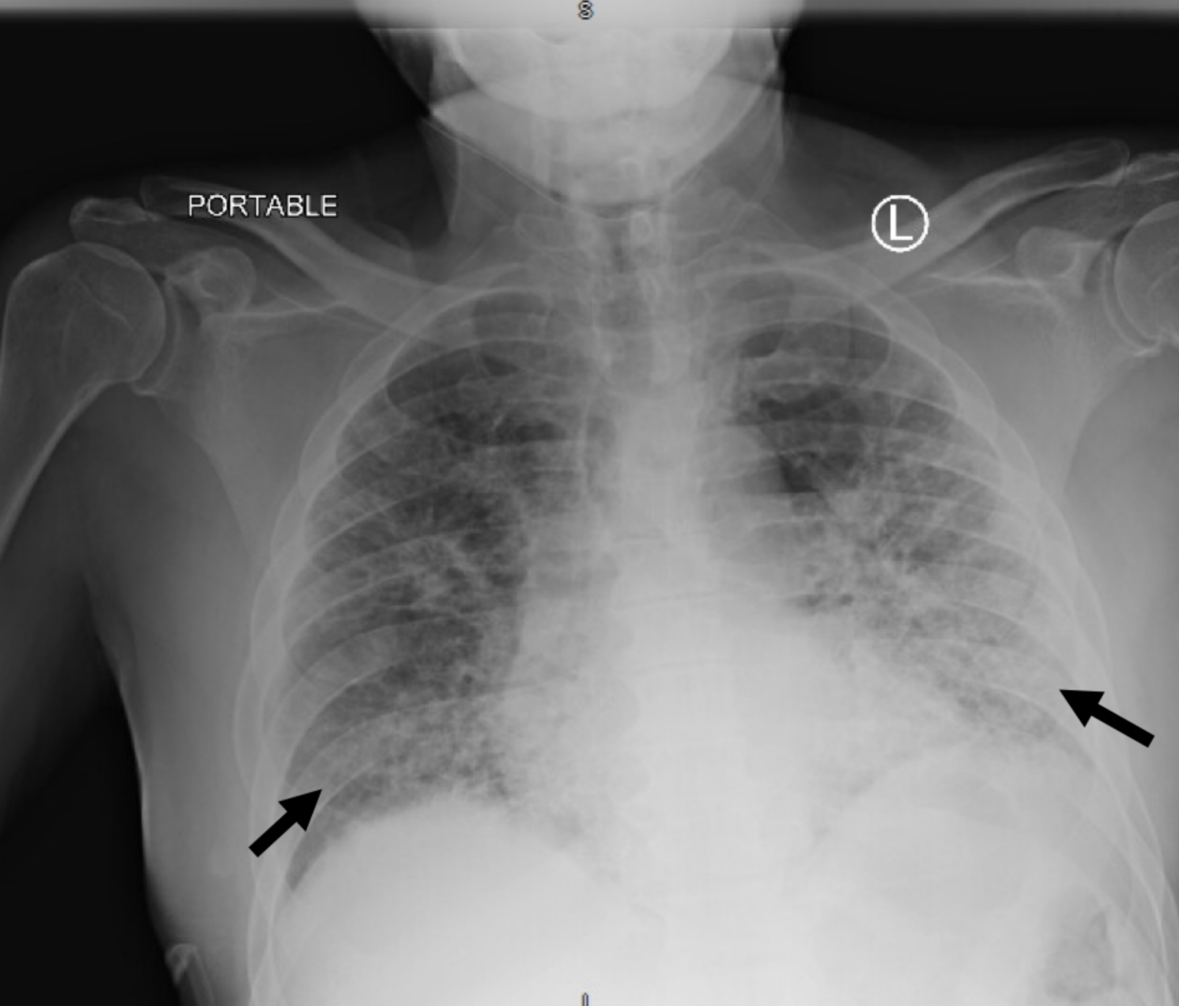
Chest x-ray showing bilateral multifocal ground-glass appearances
